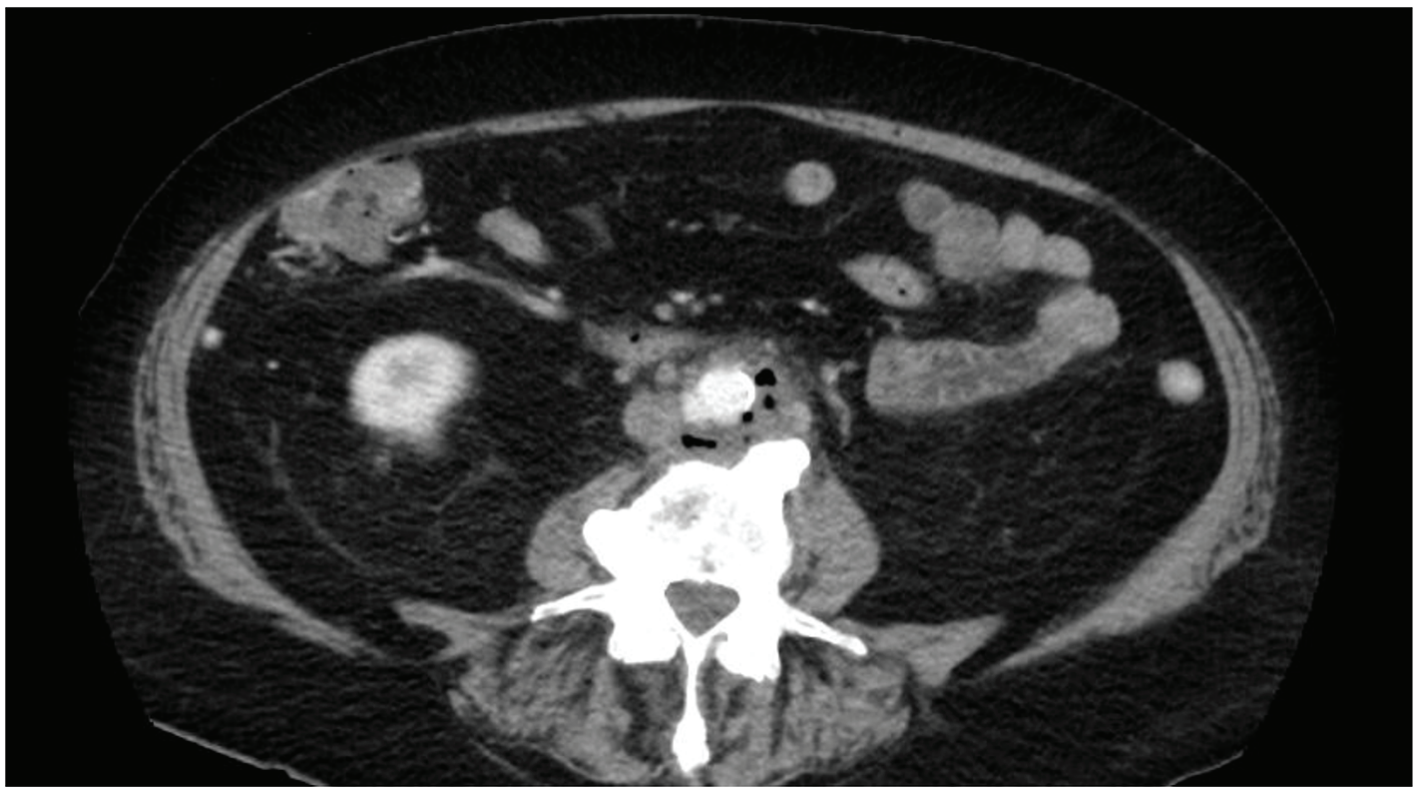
Coronal CT showing intussusception at the junction between the transverse colon and the hepatic flexure.
